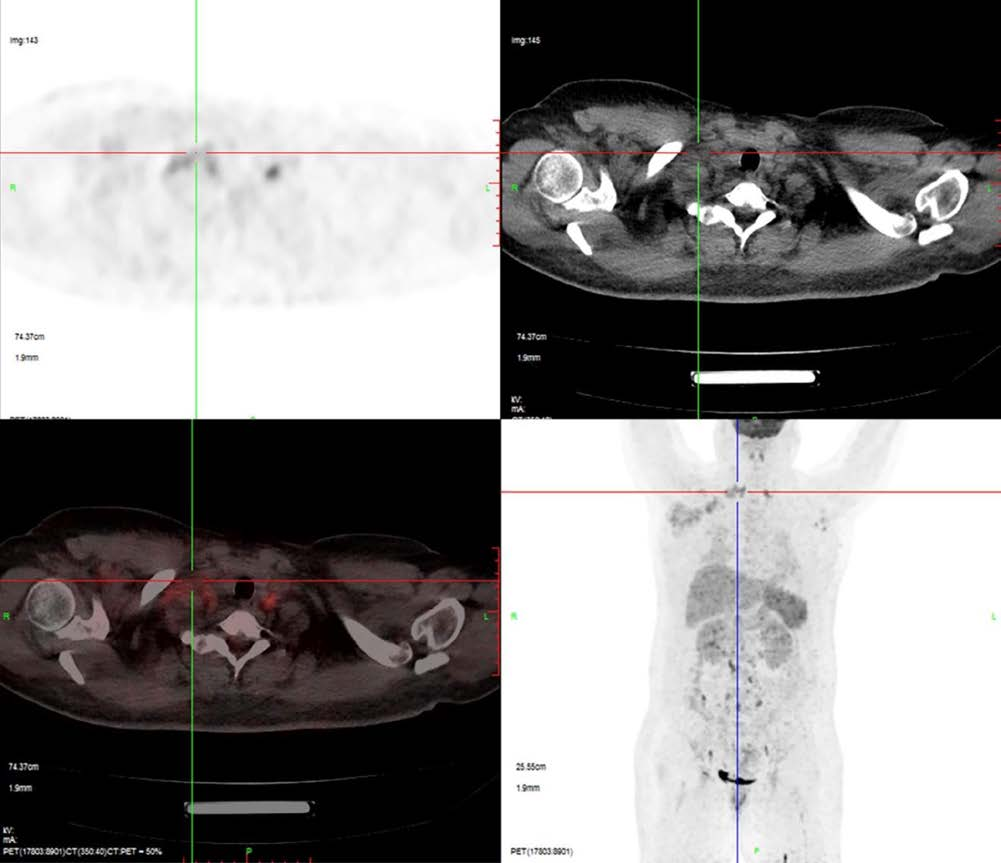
PET/CT images showing lymphomatous infiltration in liver and spleen. Whole-body positron emission tomography (PET)/CT showed that lymphoma could not be excluded, along with lymphomatous infiltration in liver and spleen. CT = computed tomography, PET = positron emission tomography.
